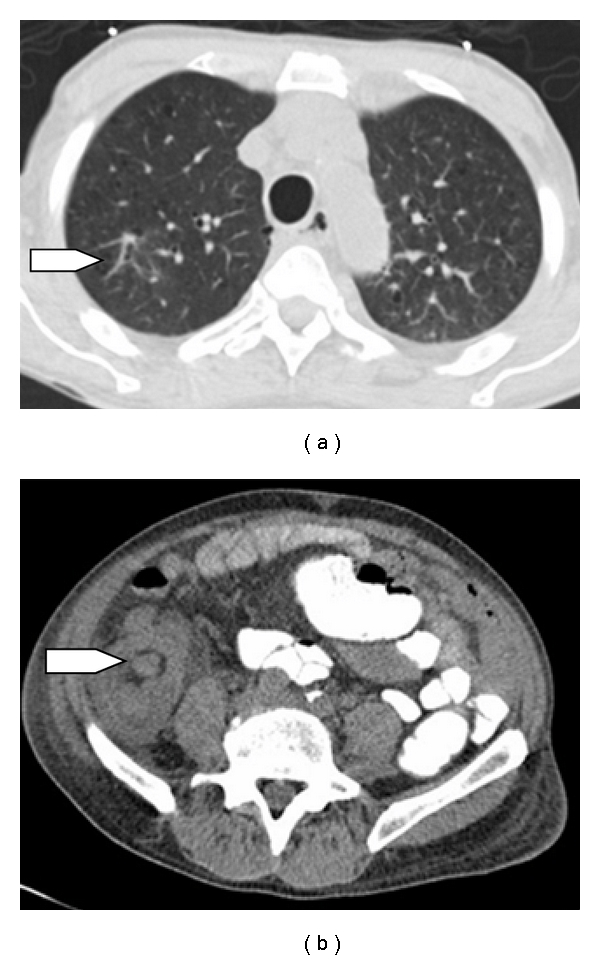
(a) A CT of the thorax revealing a spiculated right upper lobe lung mass. (b) A CT of the abdomen showing ileocecal intussusception.
